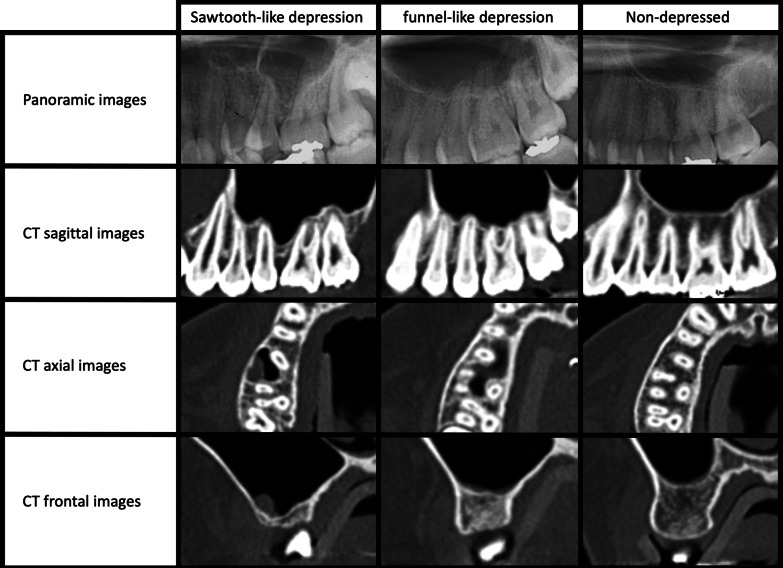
Representative panoramic images and their corresponding CT images
